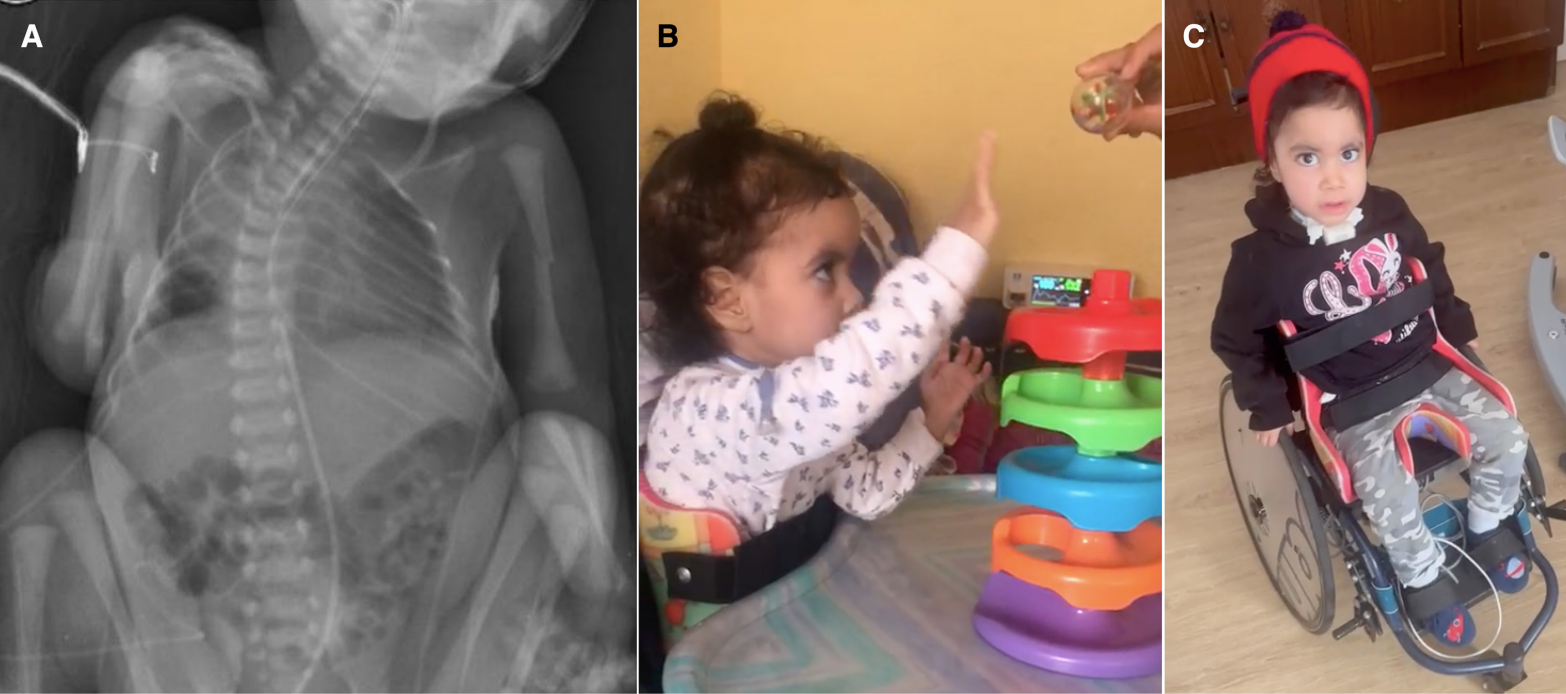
Clinical images of the two individuals with MYL1‐related congenital myopathy. (A) Thoraco‐abdominal radiograph of Individual 1 at birth, revealing a bilateral humerus fracture. The fracture is displaced on the left side and nondisplaced on the right side. (B) Lateral photograph of Individual 2 at 2 years and 4 months, demonstrating head control and the ability to elevate upper limbs against gravity. (C) Frontal photograph of Individual 2 at 2 years and 9 months, highlighting preserved strength in the upper limbs and hands compared to the lower limbs, as she is even able to propel her wheelchair.
